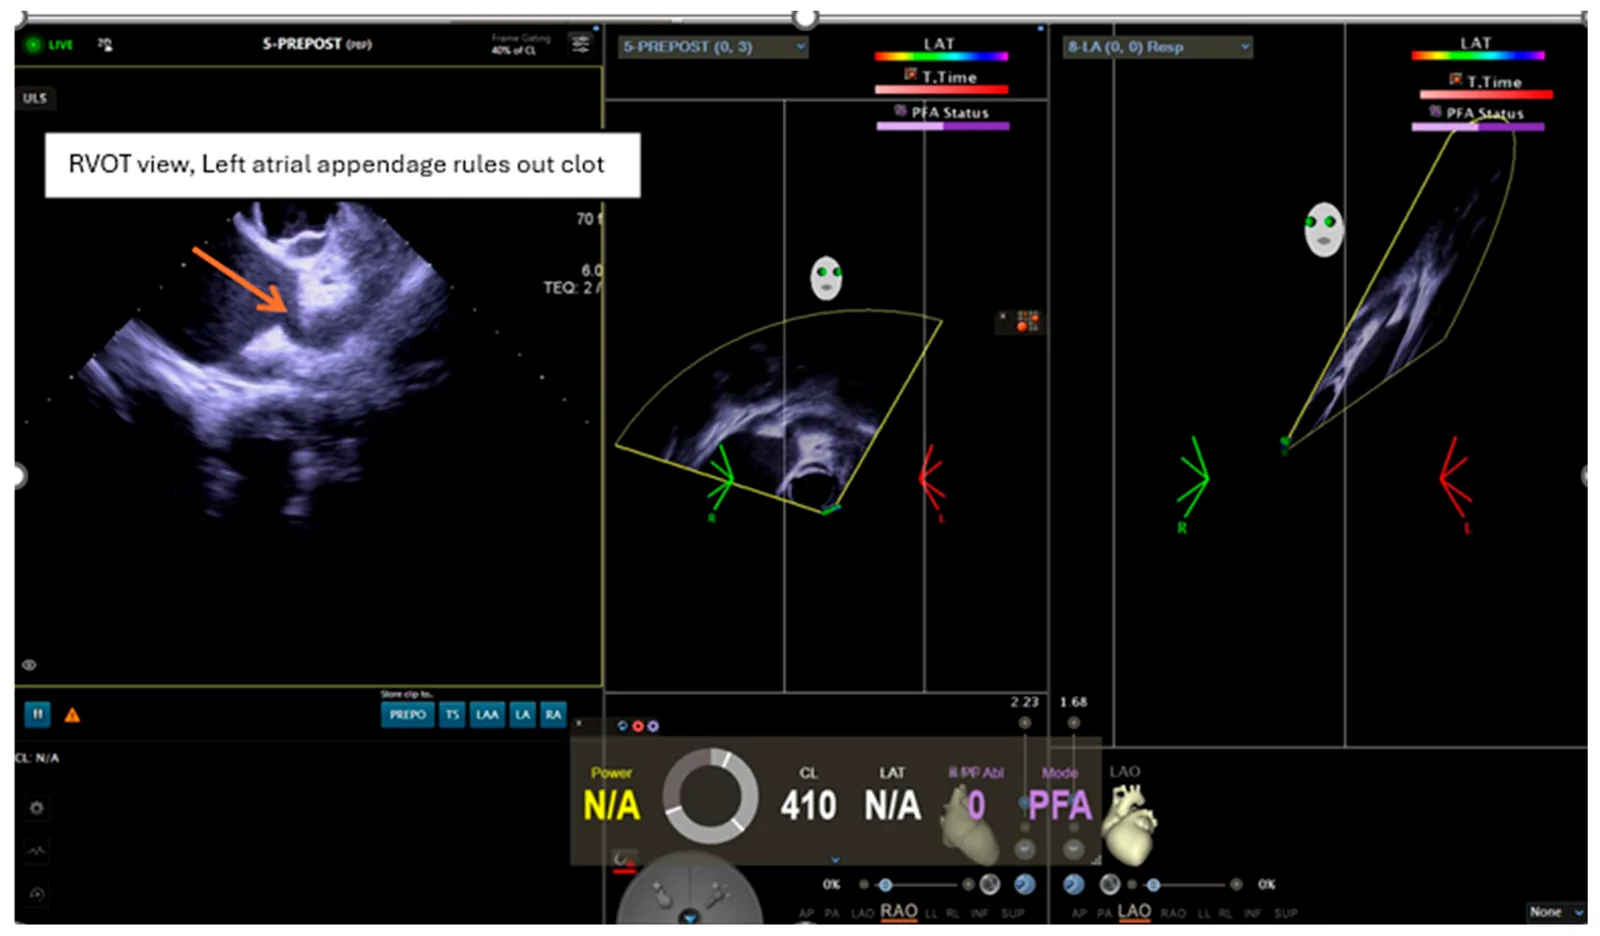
Intracardiac echocardiography (ICE) assessment of the left atrial appendage (LAA) from the right ventricular outflow tract (RVOT) view, ruling out left atrial appendage thrombus prior to ablation. The RVOT view is obtained when the standard right atrial ICE window is inadequate. This step is performed at the start of every fluoroless procedure as part of the pre-ablation safety assessment. RVOT, right ventricular outflow tract; LAA, left atrial appendage.
